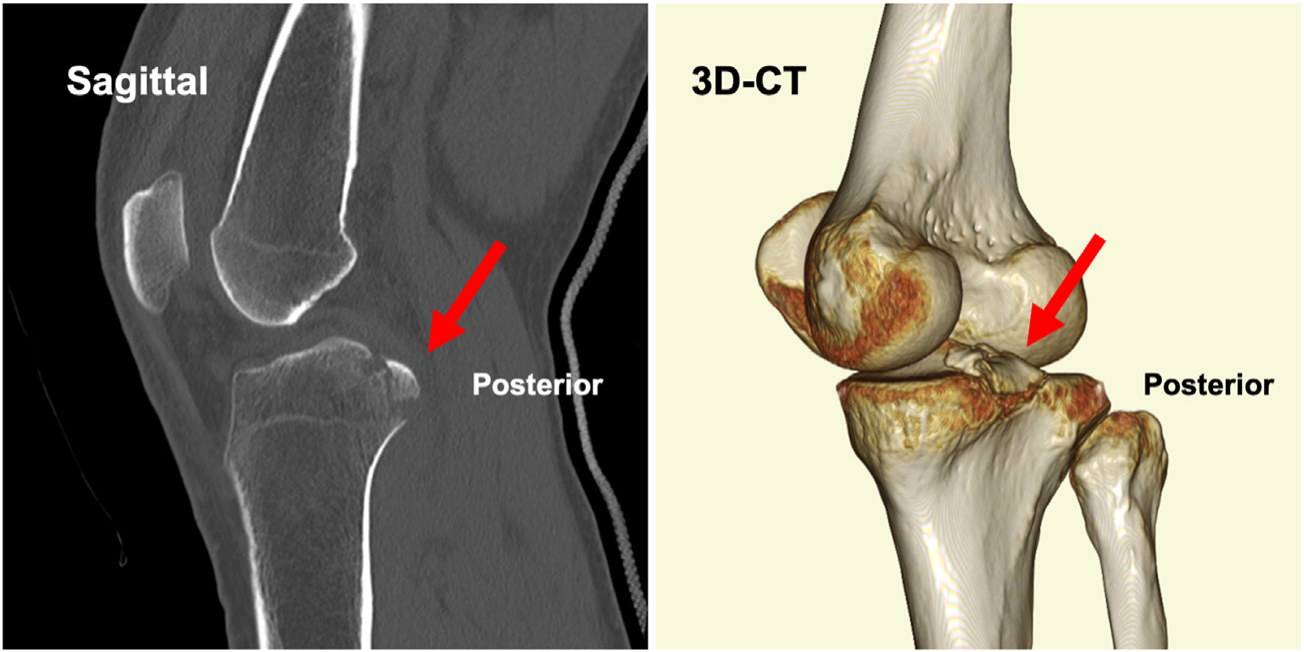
Preoperative sagittal and 3-dimensional computed tomography (3D-CT) in the right knee. A tibial avulsion fracture of the posterior cruciate ligament is observed. The main bone fragment is hinged type (arrow).
